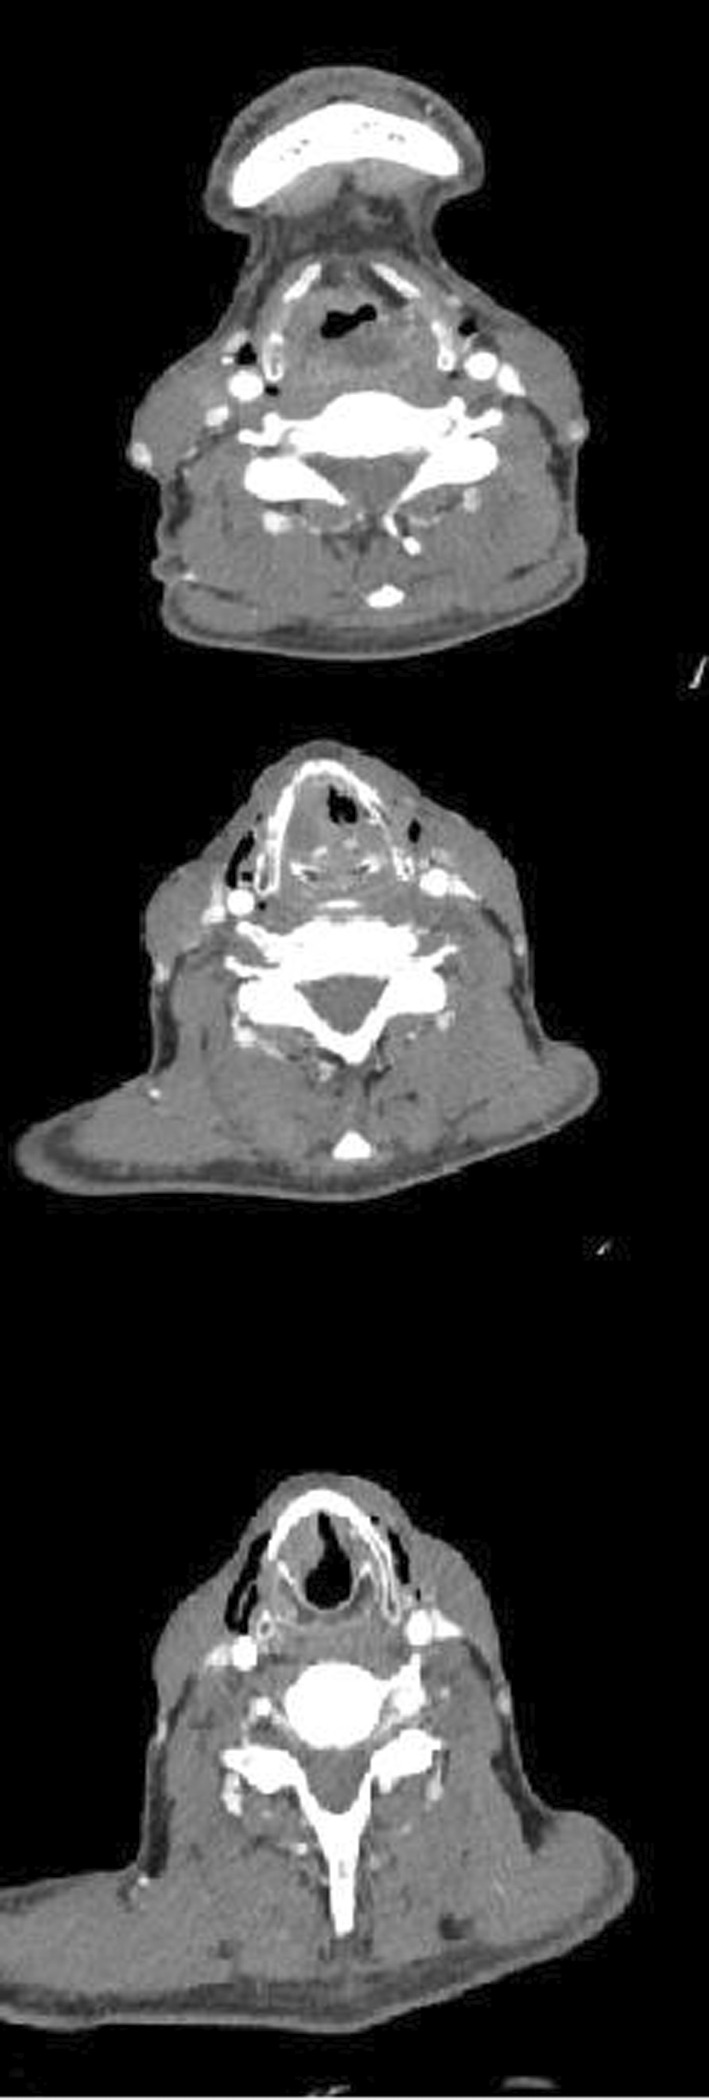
Spiral neck CT scan showed a heterogeneous supraglottic mass at midline with invasion to the left aryepiglottic fold, and inferior extension to glottis on the right side.
